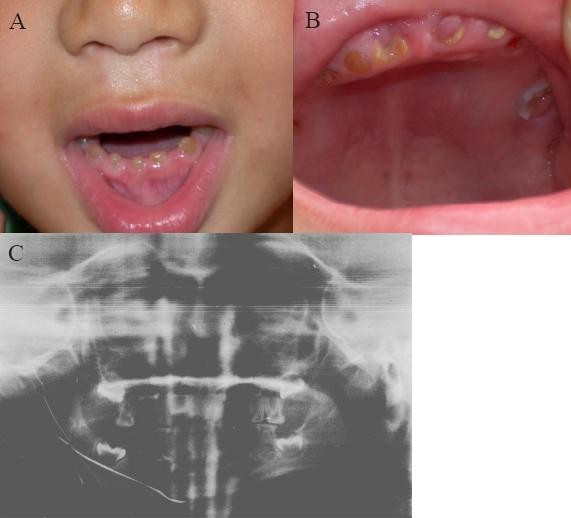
a-b. Oral photographs from the affected individual (IV:2 in Fig. 1). The primary teeth showed shade of brown and almost complete attrition of the enmel layer. c. Panorex radiographs from the affected individual (IV:2 in Fig. 1).
